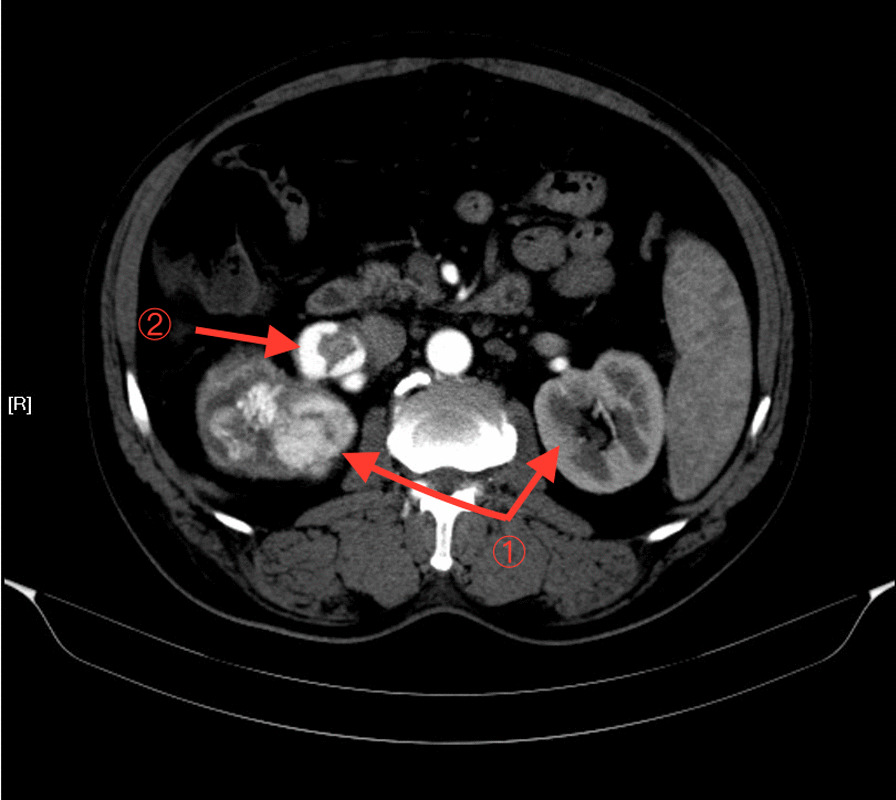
CTA showed that ① the mass in the right kidney was significantly enhanced, but that in the left kidney was not significantly enhanced; ② there was thrombosis in the right renal vein, while the left renal vein had no thrombosis
